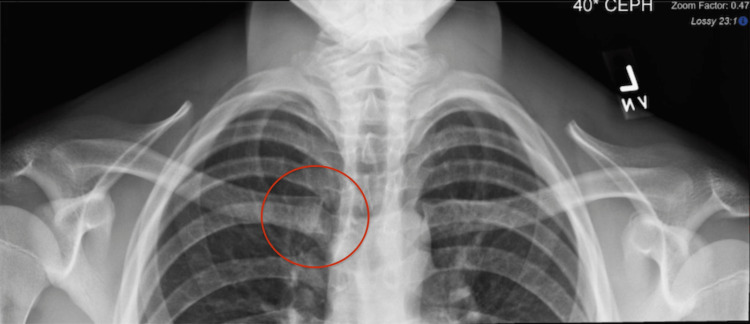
X-Ray: Anterior-Posterior View, 40 Degrees Cephalad Red circle: right sternoclavicular joint
